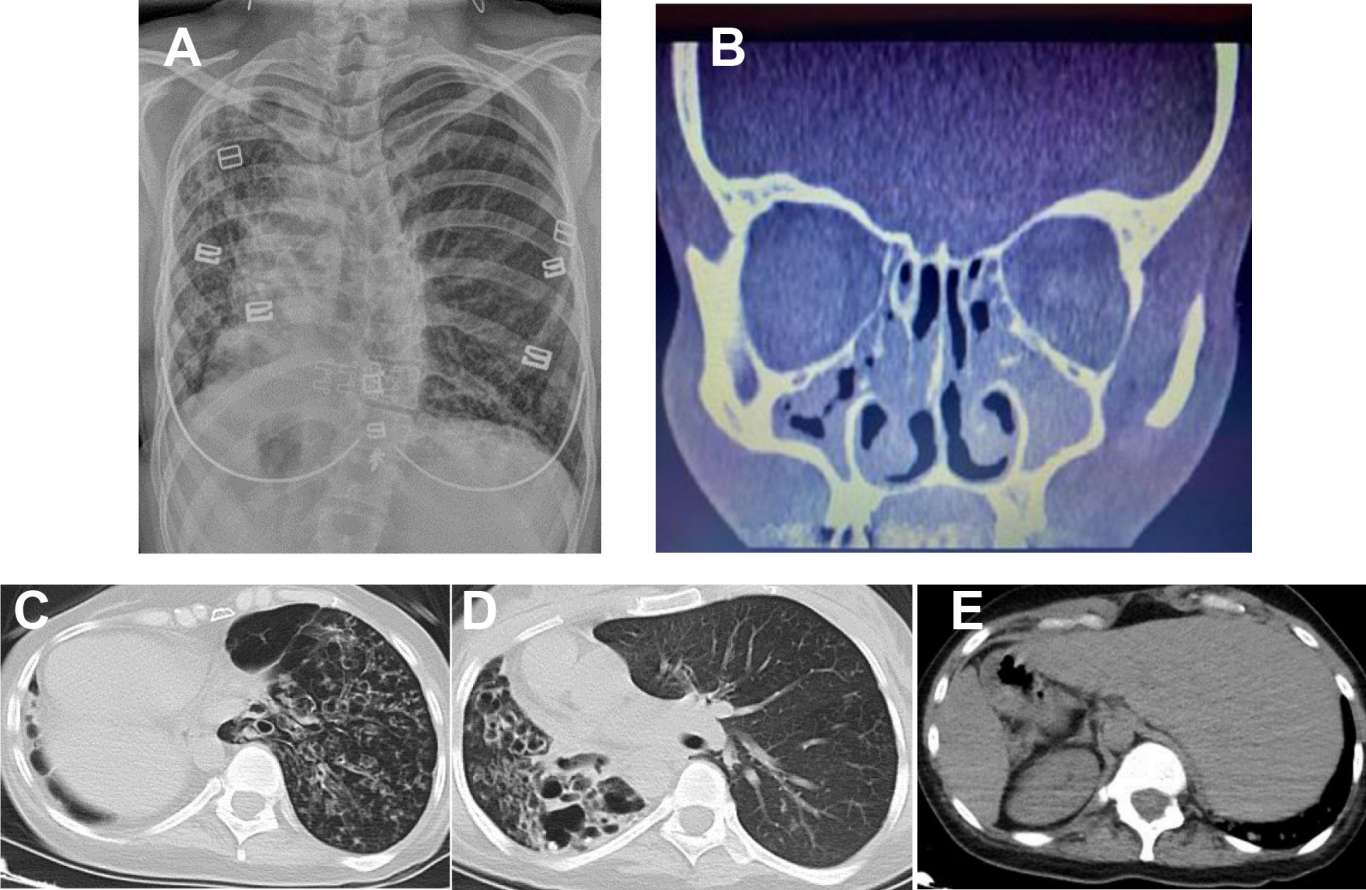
(A) Chest x-ray: heart and fundus air shadow on the right; (B) paranasal sinus tomography indicating sinusitis; (C-D) thorax tomography indicating cystic bronchiectasis in both lungs; (E) upper abdomen tomography: the spleen and stomach on the right, the liver on the left.
